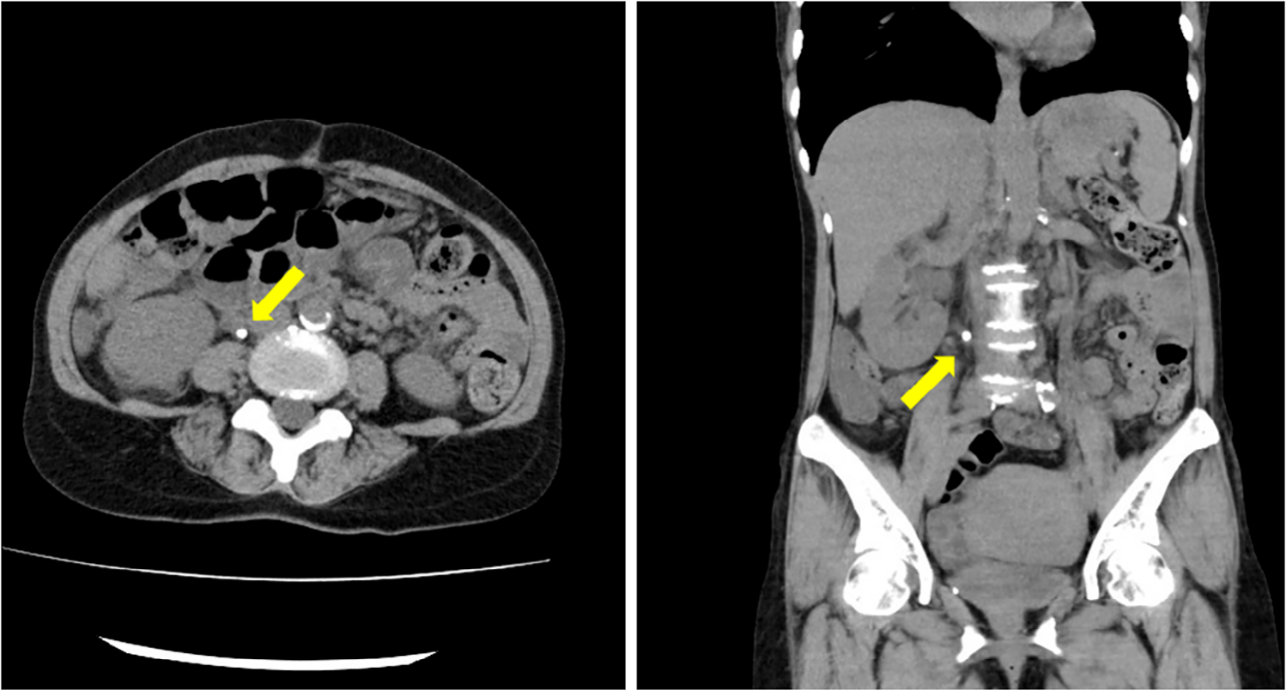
Images of abdominal computed tomography. The left figure is axial, and the right figure is the coronal view. Yellow arrowheads indicate a right ureter stone. The right ureter stone is located at the ureteropelvic junction. Right hydronephrosis is also shown in the coronal view.
